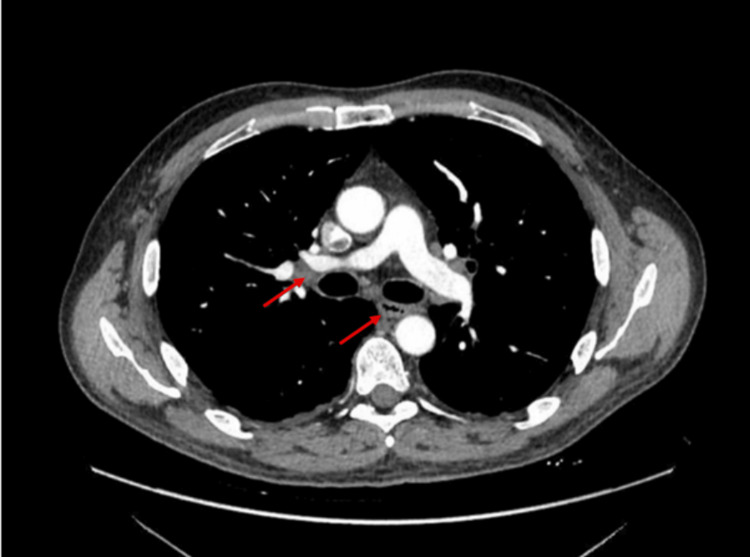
CT thorax, soft tissue windows showing mediastinal and right hilar lymphadenopathy. Arrows indicate the enlarged nodes.
